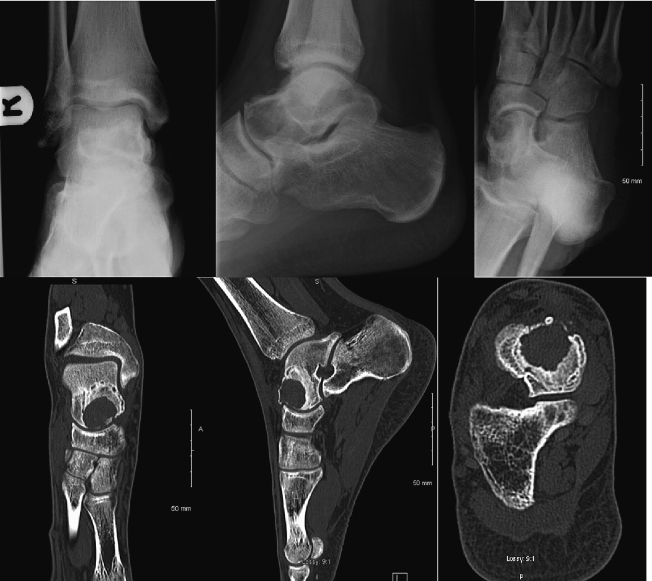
(A, B, C, D, E, F): Ankle radiographs at 4.5 months post initial curettage and grafting. There was a persistent, rounded focal lucency identified within the medial aspect of the head of the talus (2.2 cm). This lesion was non-aggressive in appearance. There was minor bone spur formation at the neck of the talus compatible with chronic anterior ankle impingement. The ankle, the subtalar, calcaneocuboid, and the bones and joints of the mid and forefoot were well preserved. Coronal, Sagittal and Axial CT images showed recurrence of a 2.2 × 2.4 cm heterogeneous cystic lesion in the head of the right talus. There was a thin sclerotic margin and a breach of the overlying cortex anteriorly and superiorly, in keeping with the previous surgical excision. There was surrounding periosteal reaction and soft tissue thickening in the dorsal aspect of the foot. No fracture was identified. Right ankle joint bones were preserved.
